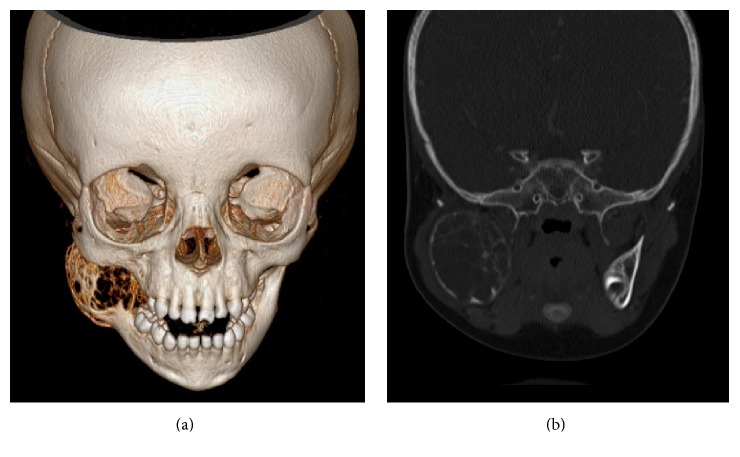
3D CT scan showing ballooning expansion of cortex.
